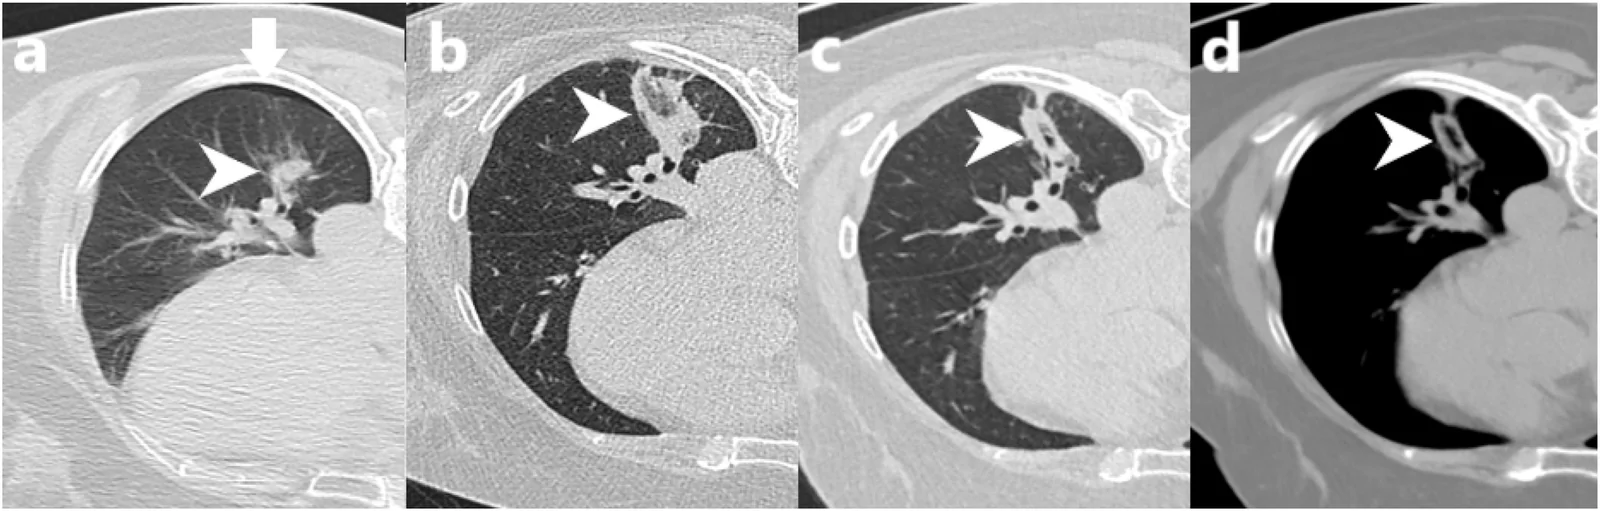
Follow-up axial chest CT (a–c), lung window; (d), mediastinal window). (a) Post-withdrawal scan showing a trace of subpleural air (arrow); the target still shows no ablation margin (arrowhead). (b) At approximately 30.5 h, a well-defined margin appears around the target zone, with ground-glass change and dense consolidation (arrowhead). (c,d) At 32 days, most of the consolidation has resorbed, forming a central cavity (arrowhead), with no pleural effusion or bronchopleural fistula.
